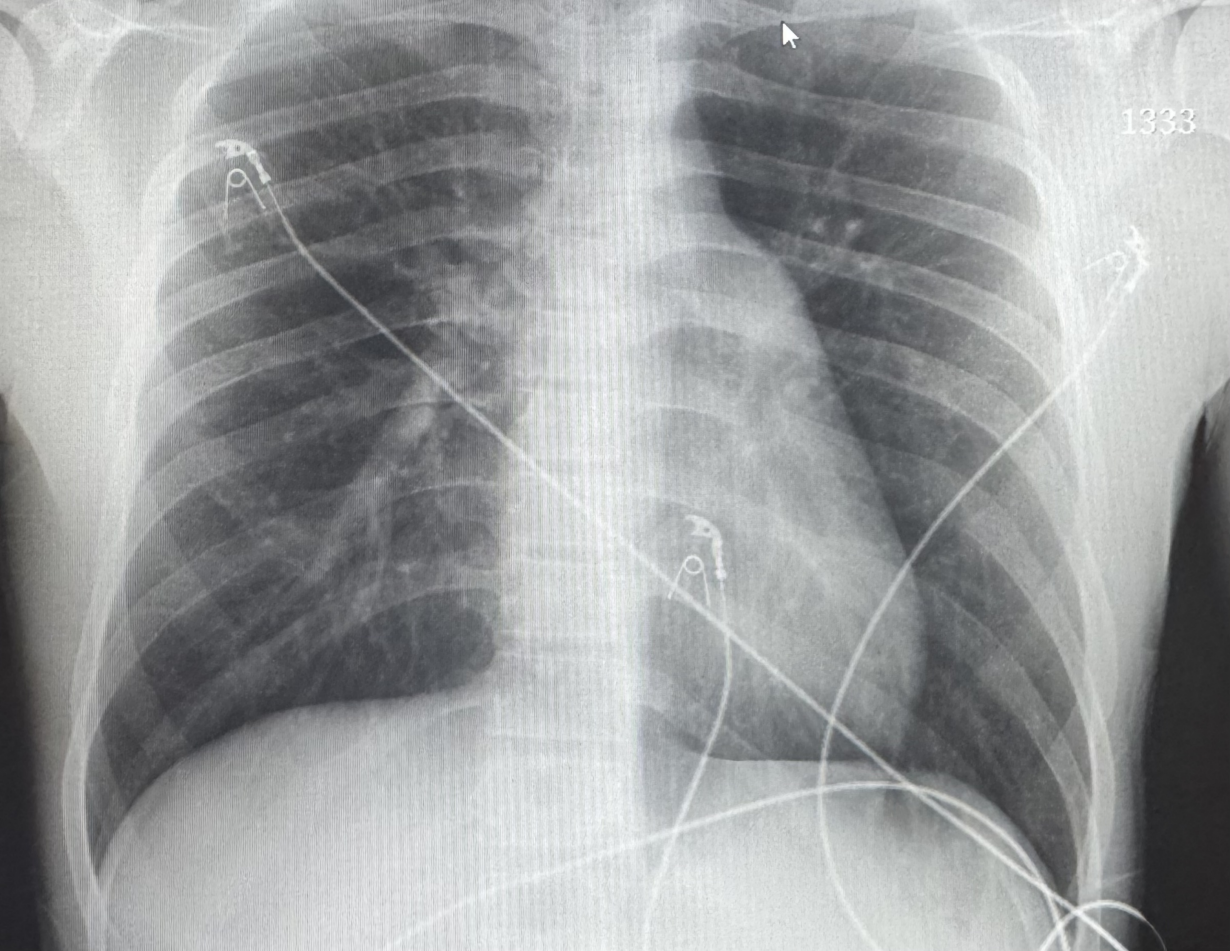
Chest X-ray from emergency department
